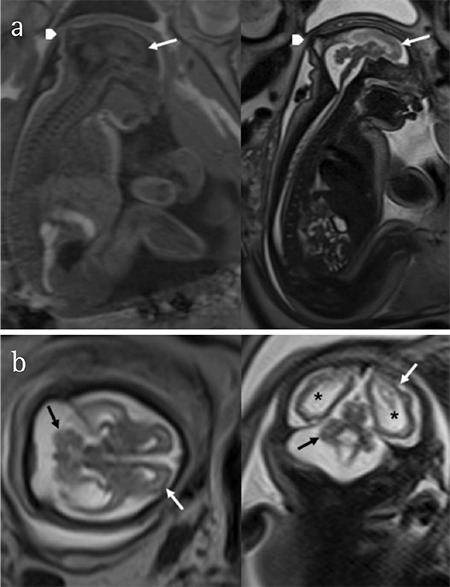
T1- and T2-wieghted magnetic resonance imaging in sagittal plane (37 weeks). Note microcephaly and smoothness of the brain surface (arrow) and redundant skin fold (arrow head) (a), axial and coronal planes showed cortical atrophy (white arrow), ventricular dilatation (*) and cerebellar hypoplasia (black arrow) (b)
